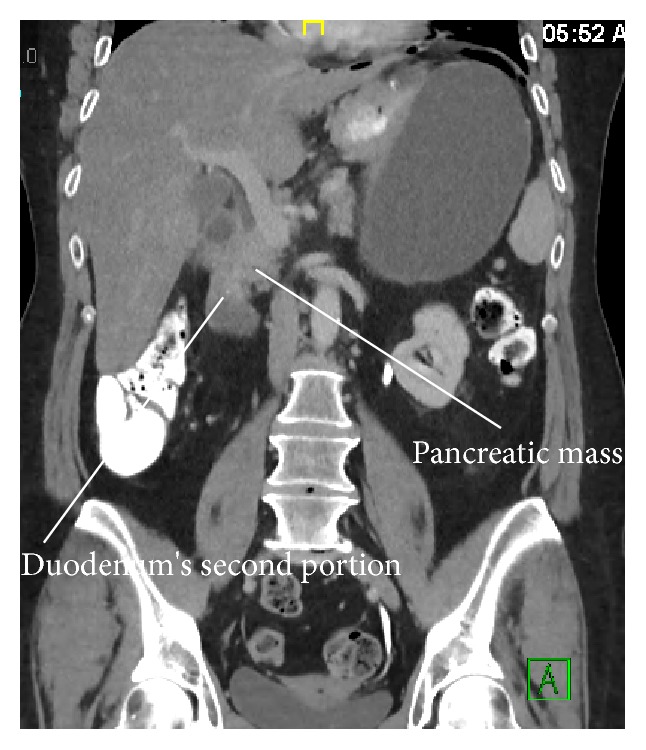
CT scan of abdomen demonstrating a pancreatic mass obstructing the second portion of the duodenum.
